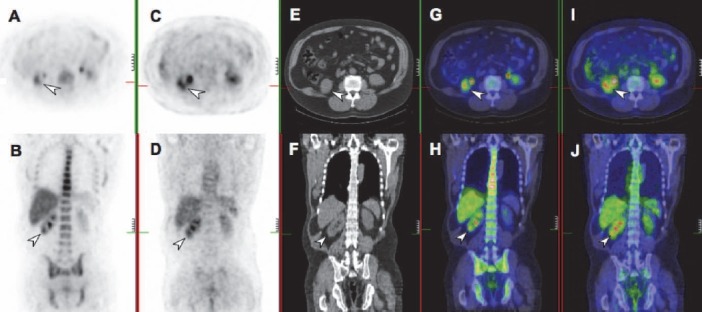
Transaxial and coronal views of a high grade clear cell RCC (arrows). Local recurrence in the right renal bed is seen on FLT PET (A, B), with focalised high uptake. The tumour is also seen in corresponding FDG PET (C, D) and CT (E, F) images. Fused PET-CT images further demonstrate the region of uptake for FLT (G, H) and FDG (I, J)
